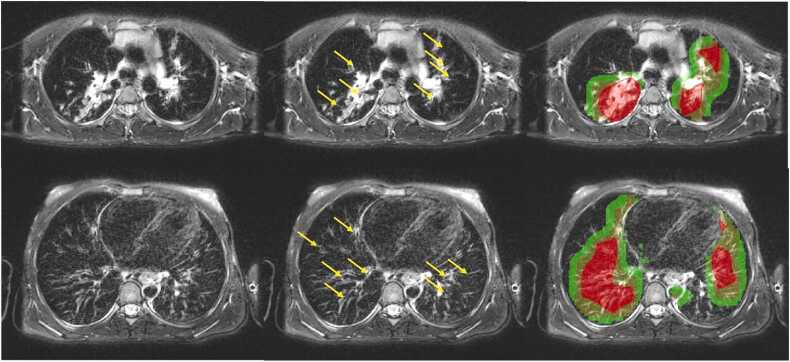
Grad-CAM visualization for bronchiectasis/wall thickening. The figure shows (from left to right): the original MRI, the MRI with highlights of bronchiectasis/wall thickening (yellow arrows) and an overlay displaying Grad-CAM. Grad-CAM relevance is color-coded, with red indicating highest relevance, yellow moderate relevance, and green lower relevance.
